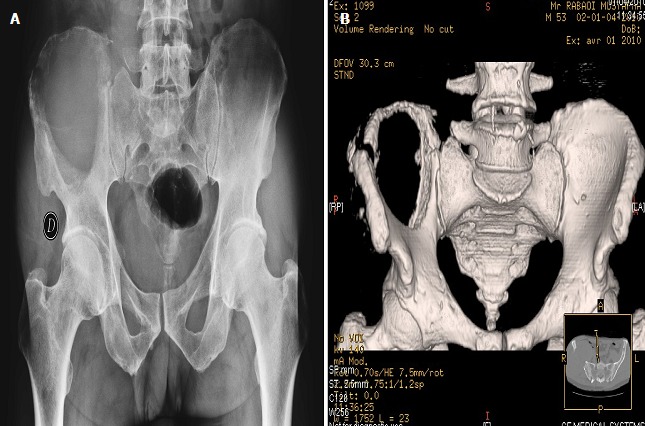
Radiographie standard du bassin de face (A) et TDM du bassin en reconstitution tridimensionnelle sans injection de produit de contraste (B): grande lacune au niveau de l'os iliaque droit avec lyse corticale
